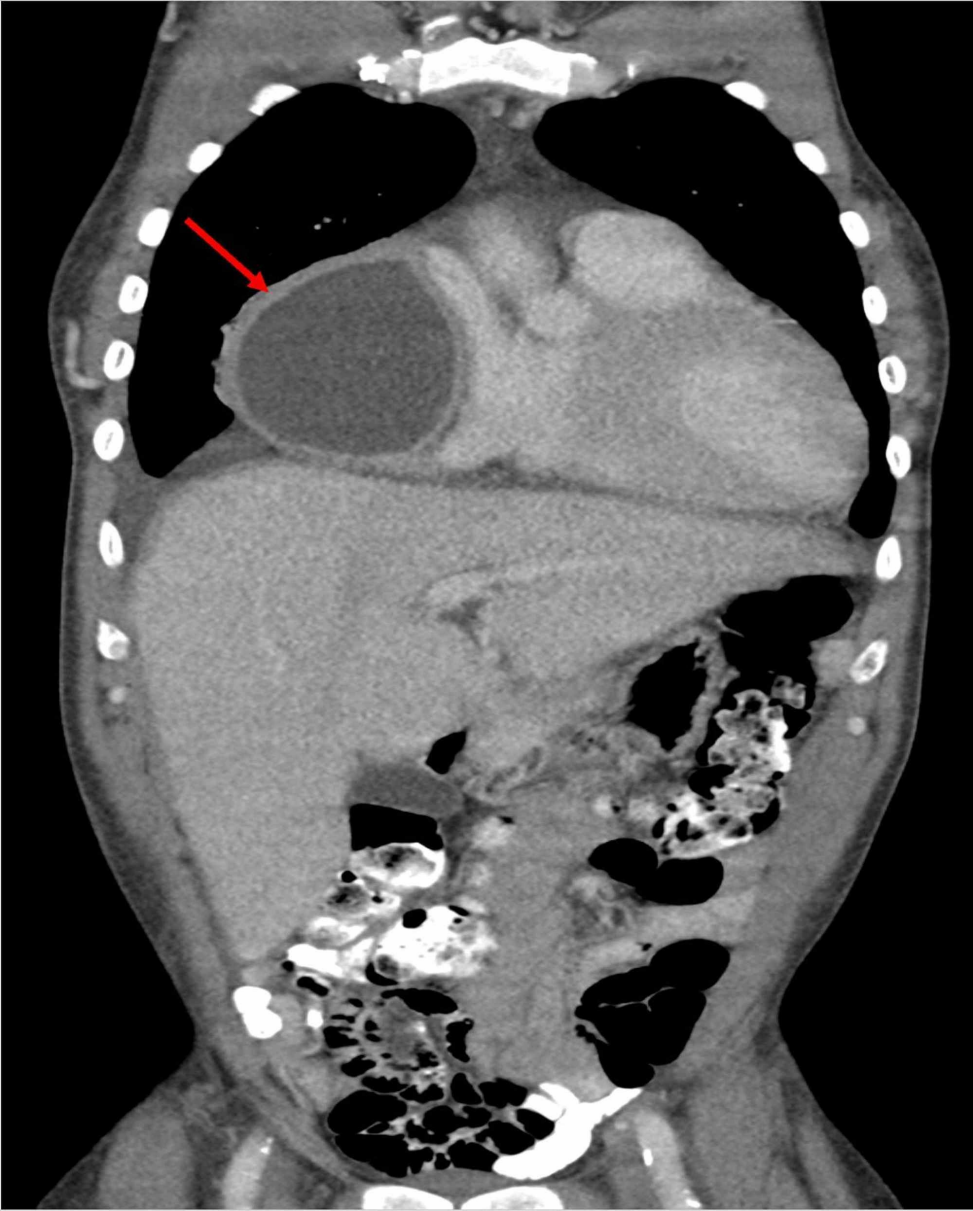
Pericardial Abscess: Coronal CT Chest, Abdomen, and Pelvis With IV and Oral Contrast.
